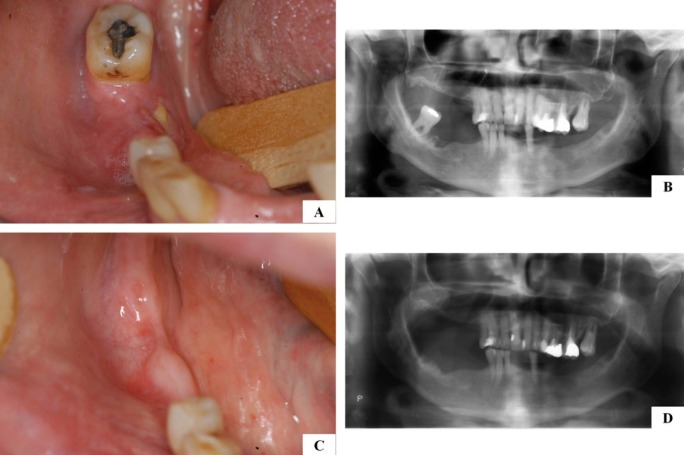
A, B) Clinical and radiographic aspects of AONJ after tooth extraction. C, D) Complete resolution
